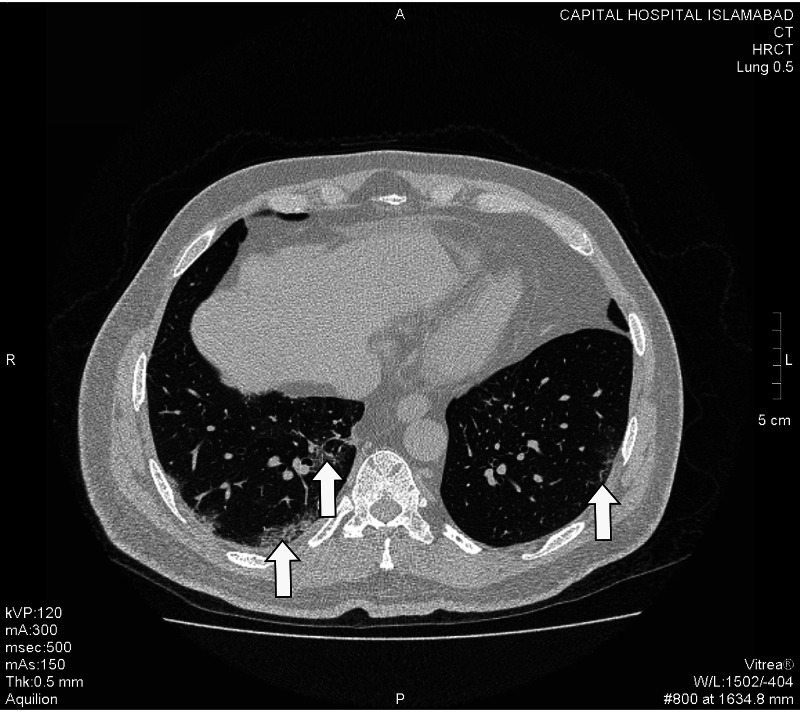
A male patient with cough and shortness of breath. HRCT demonstrates few peripheral and dorsal ground-glass opacities with a crazy-paving pattern in bilateral lower lobes (white arrows). HRCT: High-resolution computed tomography.
